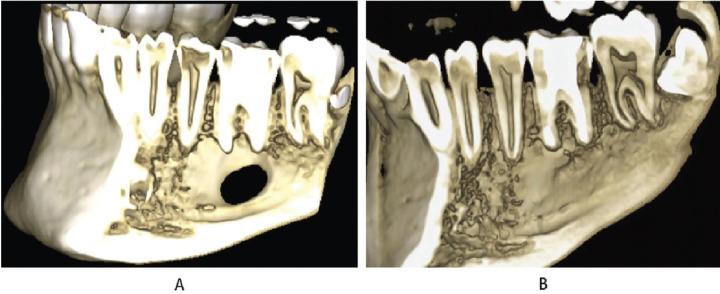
Three-dimensional reconstruction of the CBCT. A: Before the surgery. Lingual cortical loss can be detected. B: 2 years follow up showed the lingual cortical completely regenerated.
